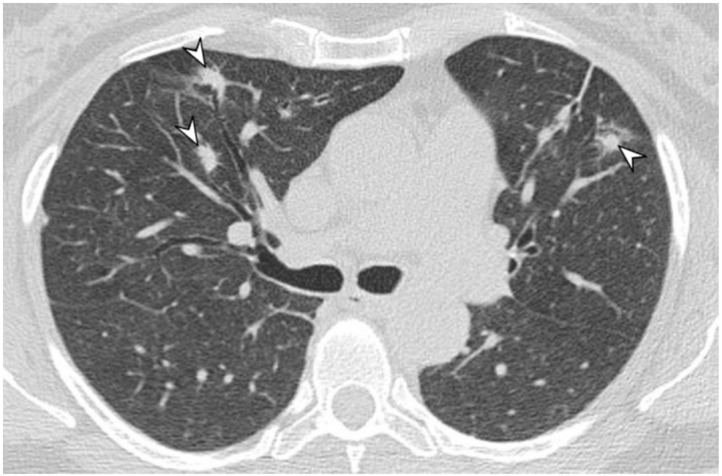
A female patient, with diagnosis of Wegener’s disease. CT image shows multiple bilateral lung nodules (arrowheads).
